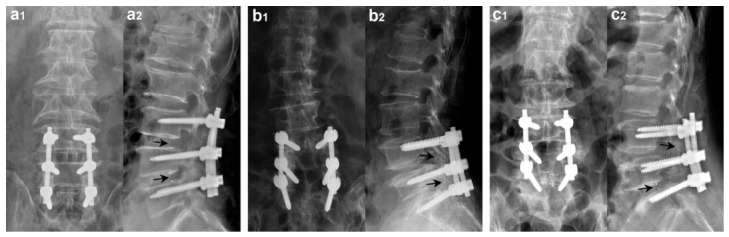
Radiographic images of 2-level lumbar fusion. (a1,a2) Postoperative anteroposterior and lateral X-ray images of posterior lumbar interbody fusion (PLIF). (b1,b2) Postoperative anteroposterior and lateral X-ray images of posterior lumbar fusion (PLF). (c1,c2) Postoperative anteroposterior and lateral X-ray images of hybrid surgery combining distal PLIF with proximal PLF.
